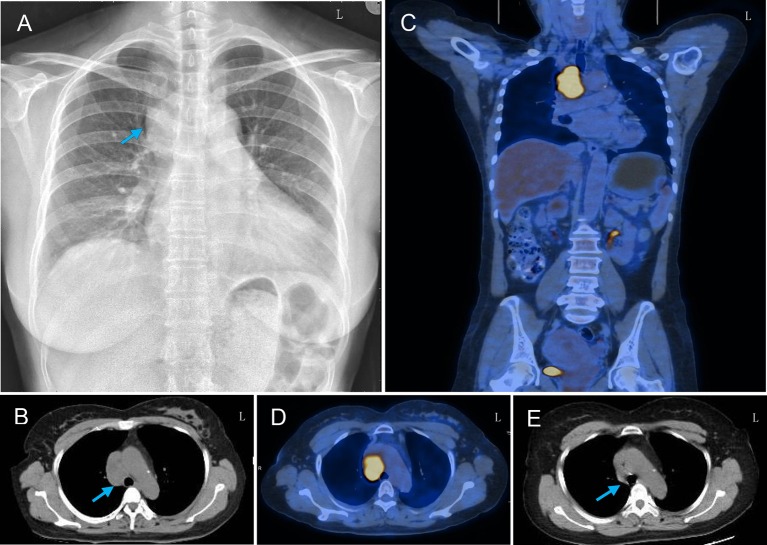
Thoracic radiograph and PET-CT findings of the patient. (A) Chest X-ray showed a round soft tissue mass (arrow) in the upper-mid mediastinum and infiltrating right lung field. (B) Thoracic CT showed a round soft tissue mass (arrow) located in the upper-mid mediastinum before the arteroae aorta. (C,D) PET-CT scan showed increased uptake of fluorodeoxyglucose signal in this mass axial and coronal plane images. (E) Repeated thorax CT showed the resolution of the mediastinal mass after chemotherapy.
